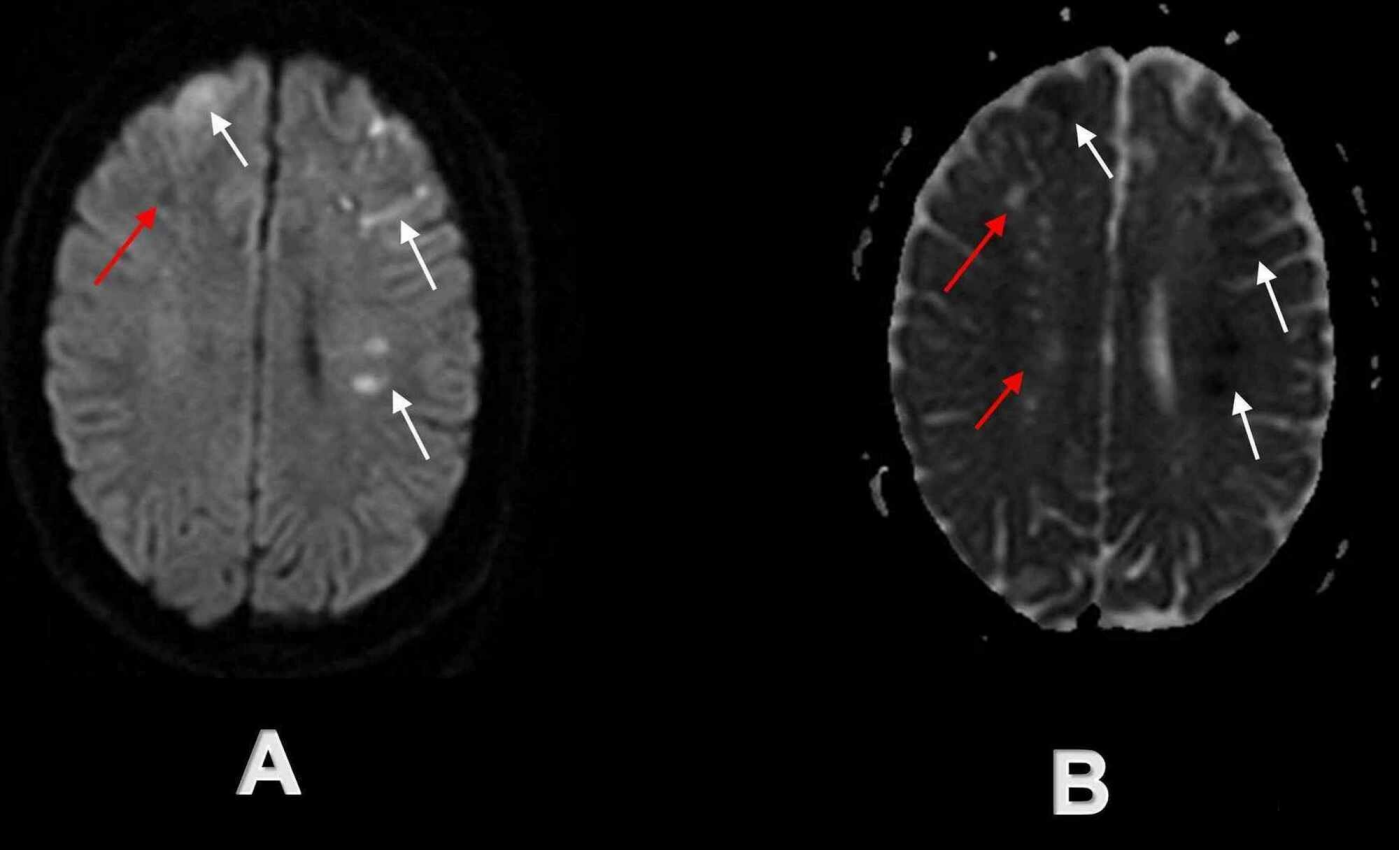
Initial brain MRI demonstrated acute and chronic ischemic infarcts in bilateral frontoparietal regions White arrows demonstrate multiple acute ischemic infarcts on axial DW sequence (A) and axial ADC sequence (B) in the bilateral frontal lobe and left parietal lobe, while red arrows demonstrate simultaneous chronic ischemic infarcts on axial DW sequence (A) and axial ADC sequence (B) in the right parietal lobe and bilateral centrum semi-ovale. DW - diffusion-weighted; ADC - apparent-diffusion-coefficient
